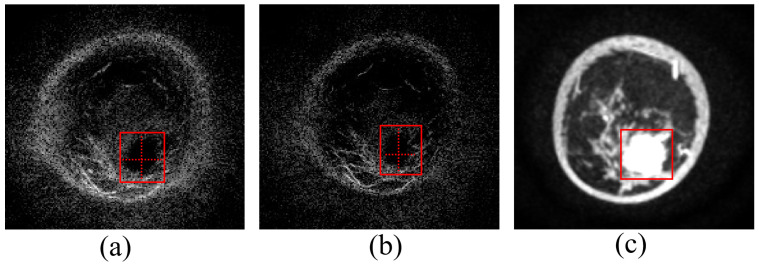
Lesion imaging: ultrasound reconstruction vs. MRI findings: (a) Ultrasound reconstruction image of the lesion at a sound speed of 1540 m/s; (b) Ultrasound reconstruction image of the lesion at a sound speed of 1513 m/s; (c) MRI findings of the lesion.
